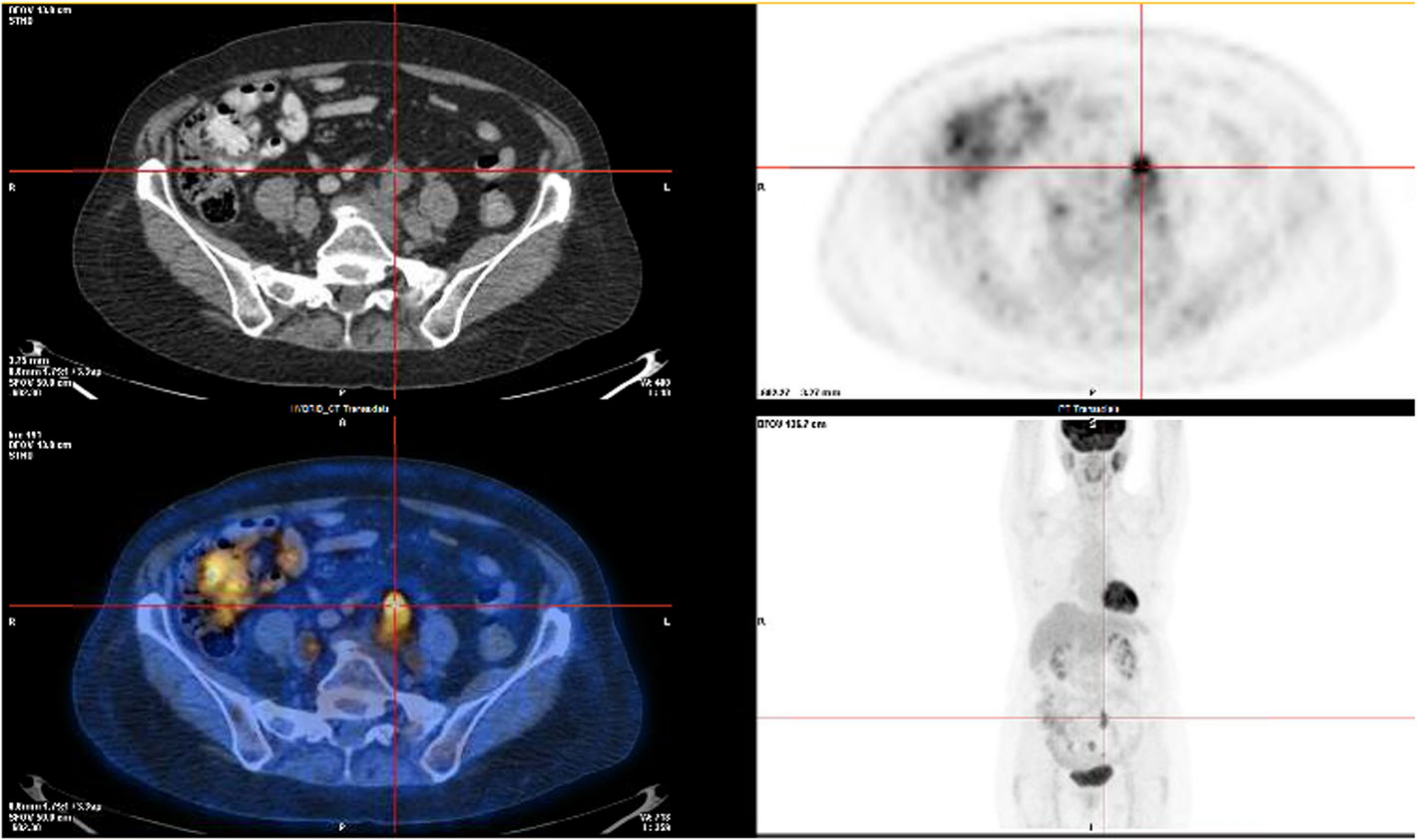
PET-CT axial and coronal images showcasing the hypermetabolic peritoneal carcinomatosis implants in the abdomino-pelvic region
